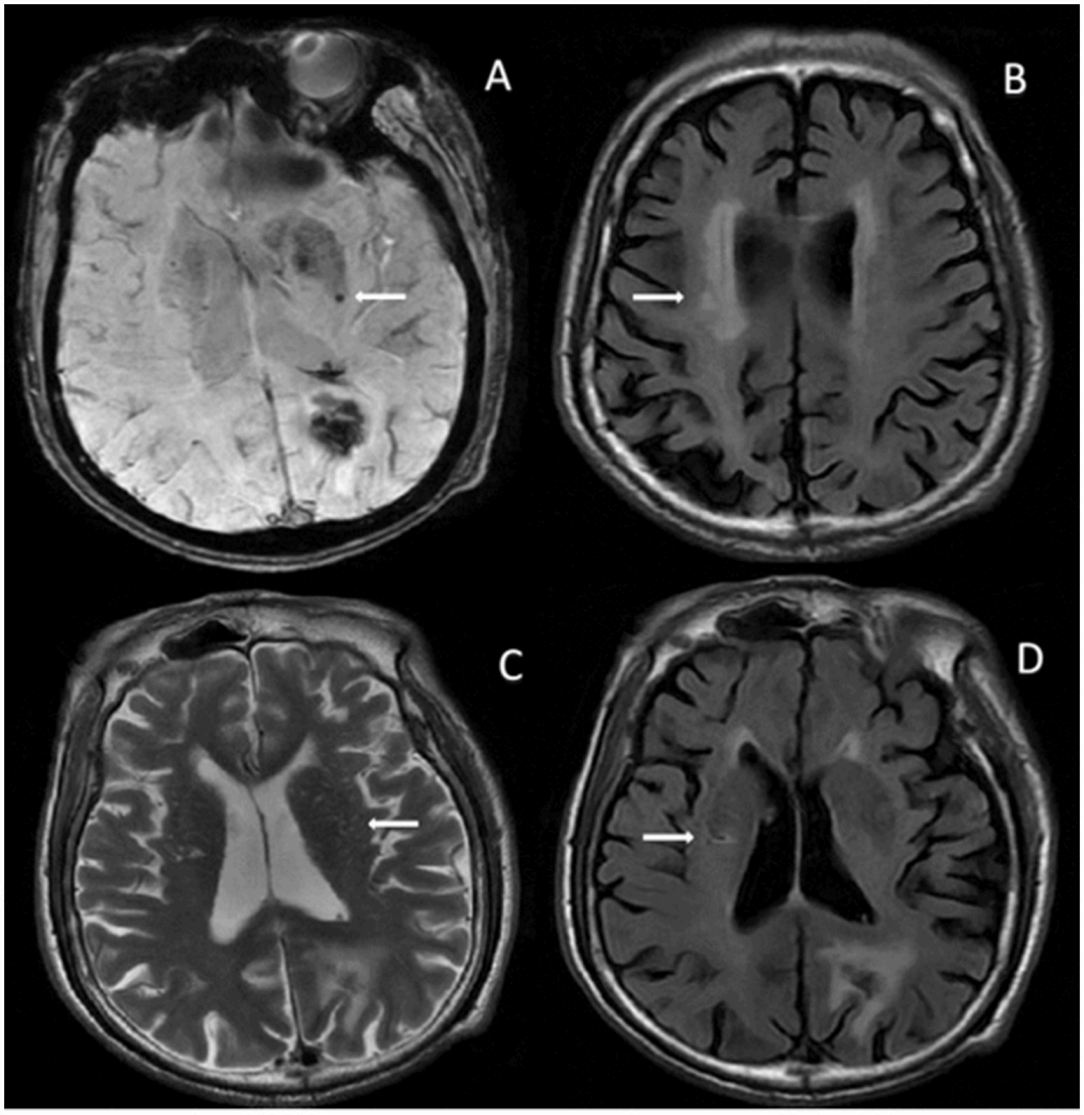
In an 80-year-old man with left occipital hematoma, MRI shows all 4 markers involved in total cSVD burden. (A) SWAN shows CMBs in left basal ganglia. (B) FLAIR image shows PWMH in bilateral side. (C) T2-sequence shows EPVS in bilateral basal ganglia. (D) FLAIR image shows lacune in right para-lateral ventricle. No R-DWIL was found on MRI (not shown). eGFR in this patient was 85.2 mL/min/1.73 m^2^, proteinuria was 2+, and cystatin C was 2.07 mg/L. CMBs, cerebral microbleeds; EPVS, enlarged perivascular spaces; FLAIR, fluid-attenuated inversion recovery; MRI, magnetic resonance imaging; SWAN, star weighted angiography; PWMH, periventricular white matter hyperintensity; R-DWIL, remote DWI lesions; eGFR, estimated glomerular filtration rate.
